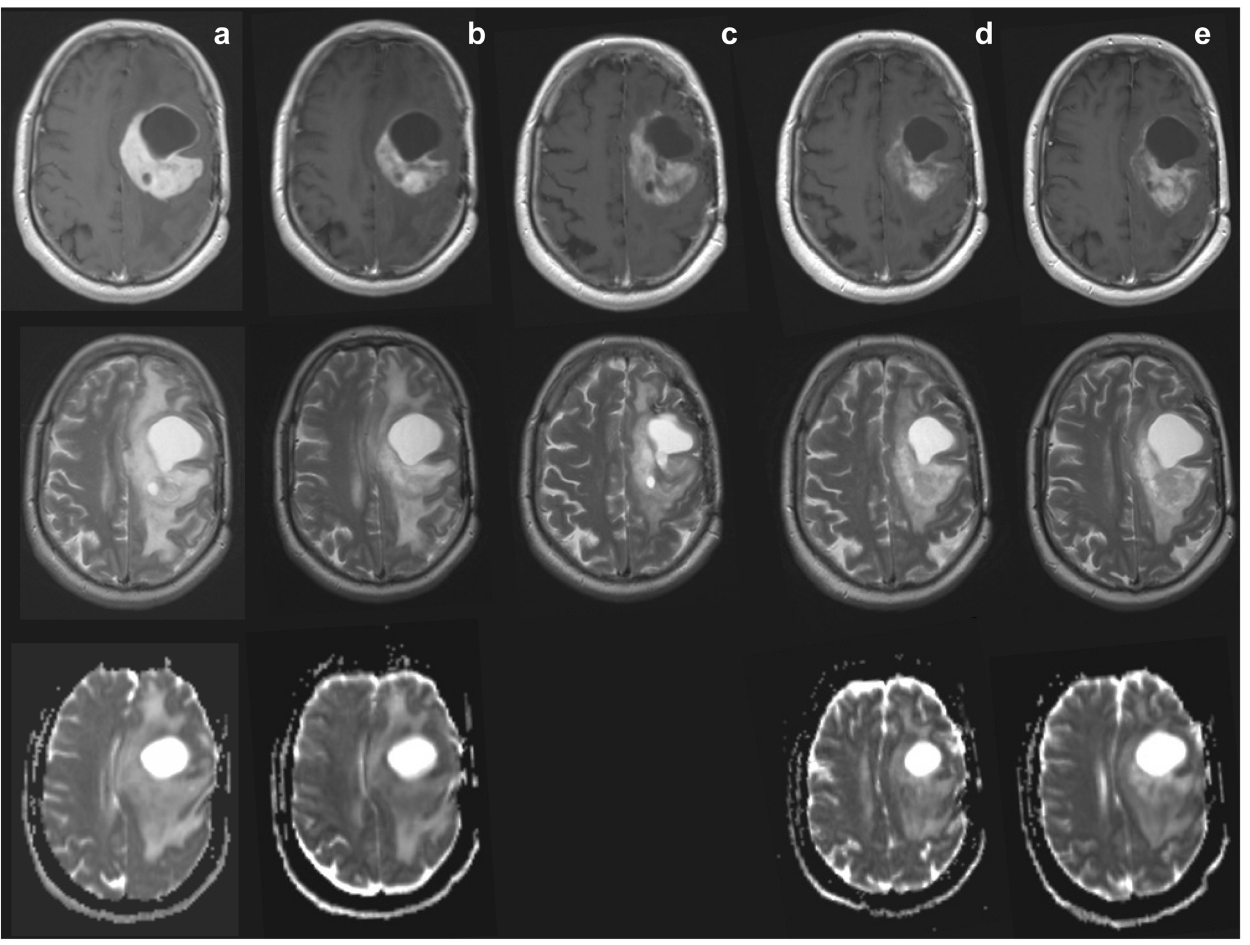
MRI scans of recurrent GBM treated with bevacizumab. MRI scans of a typical patient with recurrent GBM, treated with bevacizumab 10 mg/kg every 3 weeks plus daily temozolomide 50 mg/m^2^. Top row T1; middle row T2; bottom row ADC (apparent diffusion coefficient. ADC c is lacking). Column (A) scans pre-treatment, showing cystic and tumour component, large midline shift, and large vasogenic oedema. Column (B) 3 days after start, showing reduced contrast enhancement, and slightly reduced midline shift. Column (C) 21 days after start, showing reduced contrast enhancement but a larger size (no progression based on Macdonald criteria), reduced midline shift, and reduced oedema. Column (D) 88 days after start, showing decreased size of tumour and cystic component, stable reduction of contrast enhancement, normalised midline shift, and slight increase of oedema. Column (E) 188 days under treatment, showing increased tumour size and cystic component, increased midline shift, and increased oedema (also in the other hemisphere).
